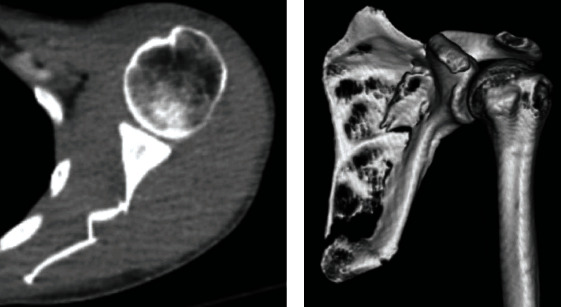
Standard CT and CT reconstruction image demonstrating more extensive left scapular body fracture.
